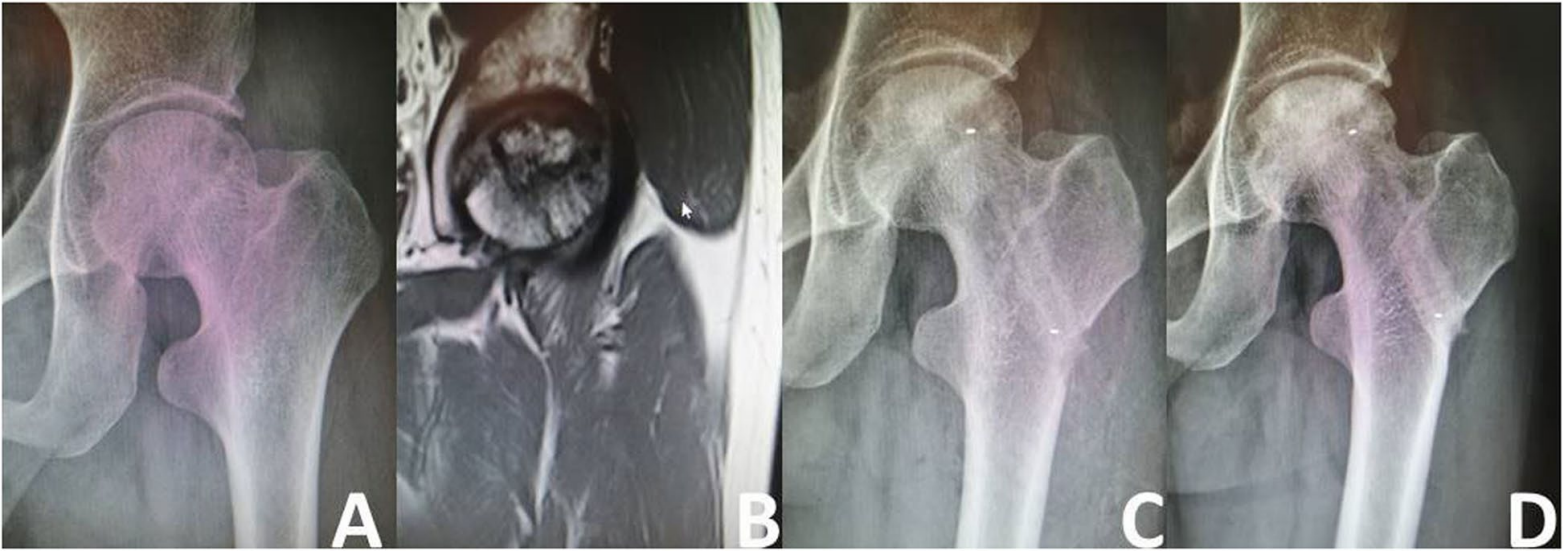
Representative case of stable osteonecrosis of the femoral head. A Preoperative radiograph. B Preoperative MRI. C Radiograph taken on postoperative day 3. D Radiographs taken 2 years postoperatively
